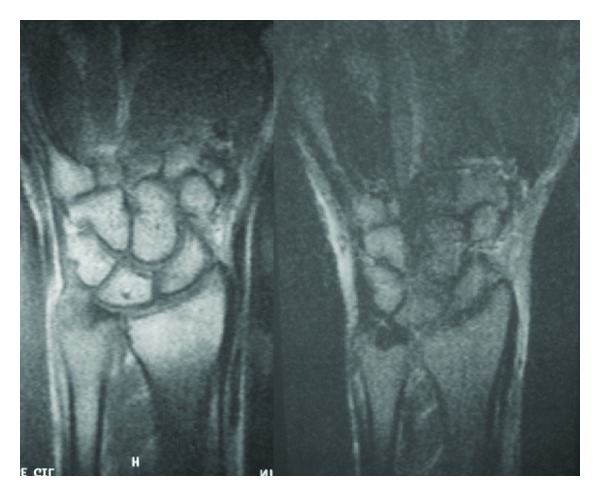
Wrist magnetic resonance scan where the T2-weighted sequence revealed a high-hyperintense signal in area of the triangular fibrocartilage and synovium. It did not demonstrate the characteristic nidus of osteoid osteoma.
